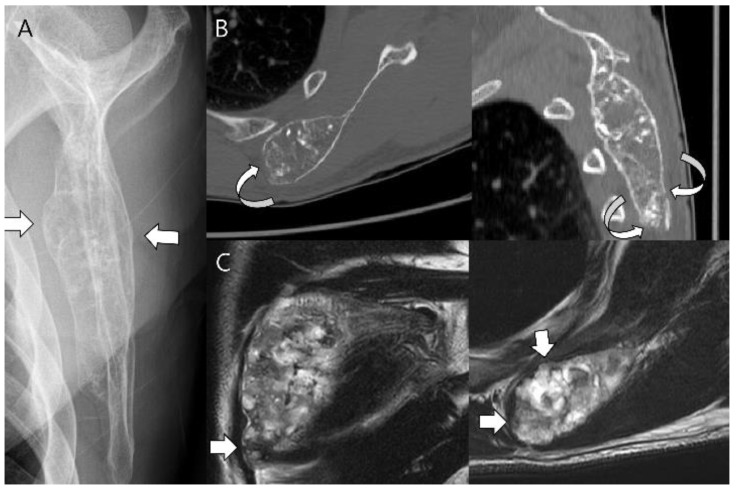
Chondrosarcoma grade 2 of the scapula in a 58-year-old man. (A) Plain radiograph shows a lobulated intramedullary mass with chondral-type mineralization and bone expansion (arrows) in the scapular body. (B) Axial and sagittal CT scans show a large intramedullary mass with cortical destruction (curved arrows). (C) Coronal and axial T2-weighted images show focal extraosseous soft tissue masses (arrows).
